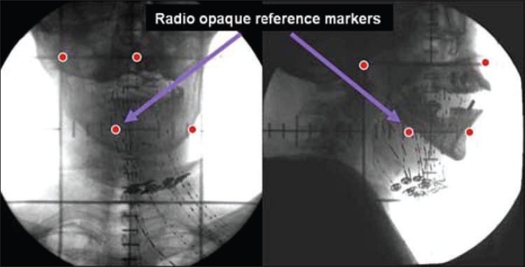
Orthogonal radiographs with X-ray dummy's and radio opaque reference markers
